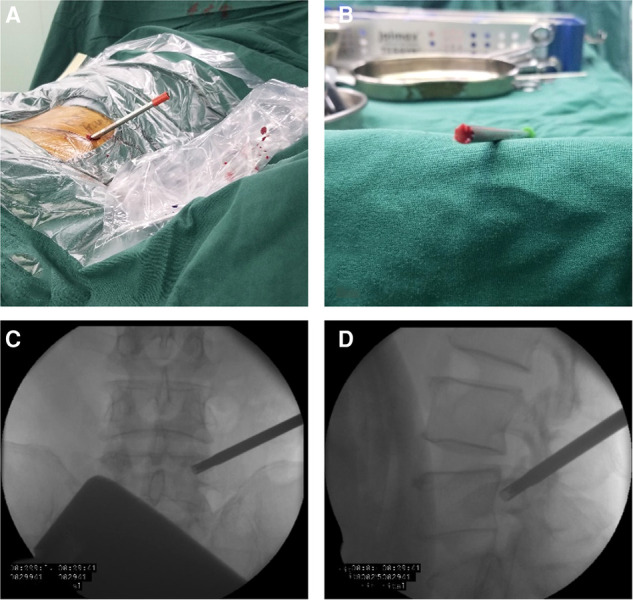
(A) The pedicular bone around the Kirschner wire is sawn by the trephine. (B) Bone can be brought out by the trephine. (C) The medial wall of the pedicle is the safety margin in the anteroposterior view. (D) The lateral view of the radiograph shows the position of the trephine.
